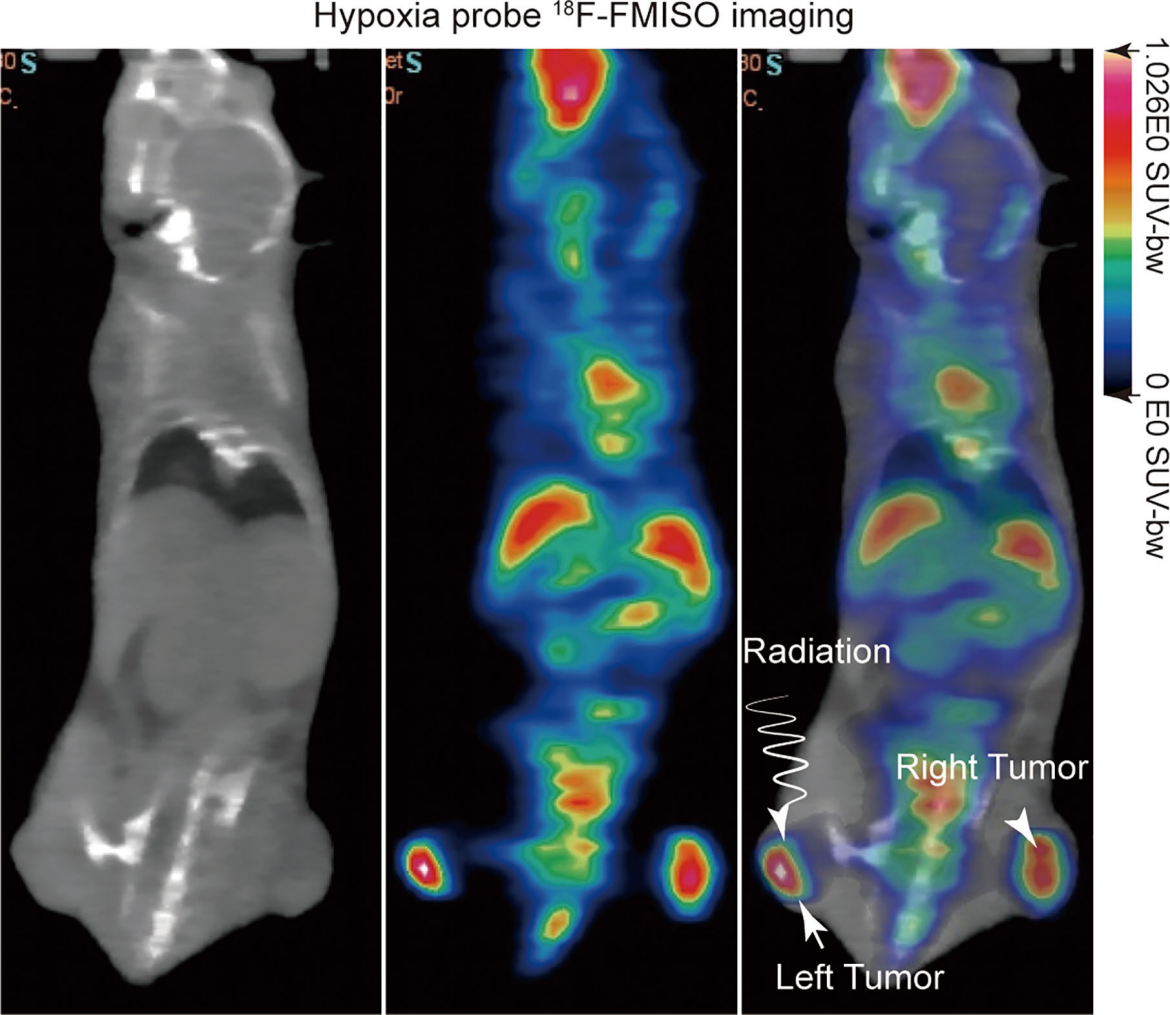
^18^F-FMISO PET/computerized tomography (CT) imaging was performed before irradiation treatment for evaluating the hypoxia status of tumors.
